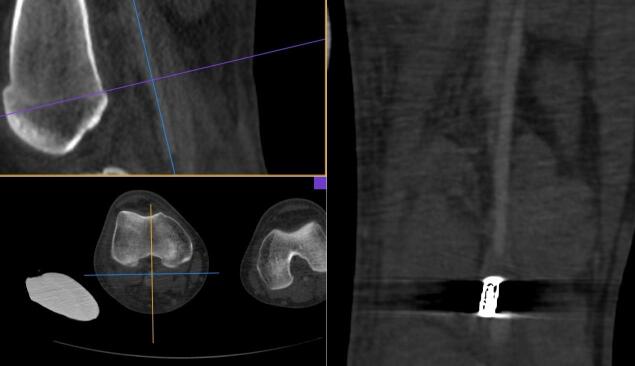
Computed tomography angiography images showing the bullet inside the arterial lumen, in the topography of the P2 segment of the popliteal artery.
